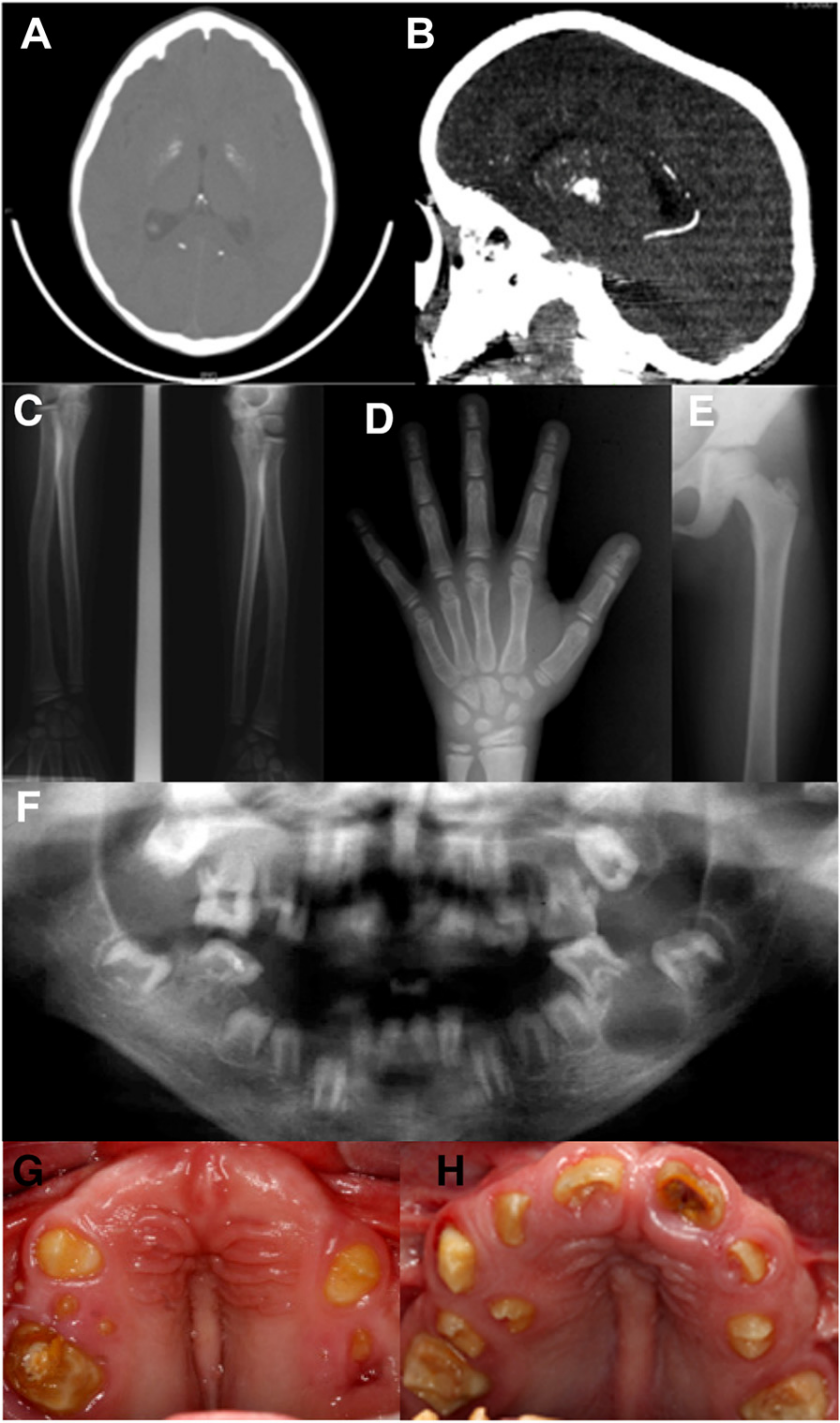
Radiographic and oro-dental features of Family 2. CT scanning identified (A) intracranial calcifications and (B) probably vascular calcifications in patient VI-1. Radiographs show (C) undermineralised long bones, (D) carpal bones and phalanges (E) and a mild radius bowing in patient VI-2. (F) Dental radiograph showing an absence of density differences between enamel and dentin, incomplete root formation and enlarged pulp chambers. Apical radiolucencies associated with permanent teeth are also present. (G & H) Affected family members presented permanent erupted teeth with yellow discoloration, hypomineralised and hypoplastic enamel. Severe delays in permanent tooth eruption were observed in both siblings.
